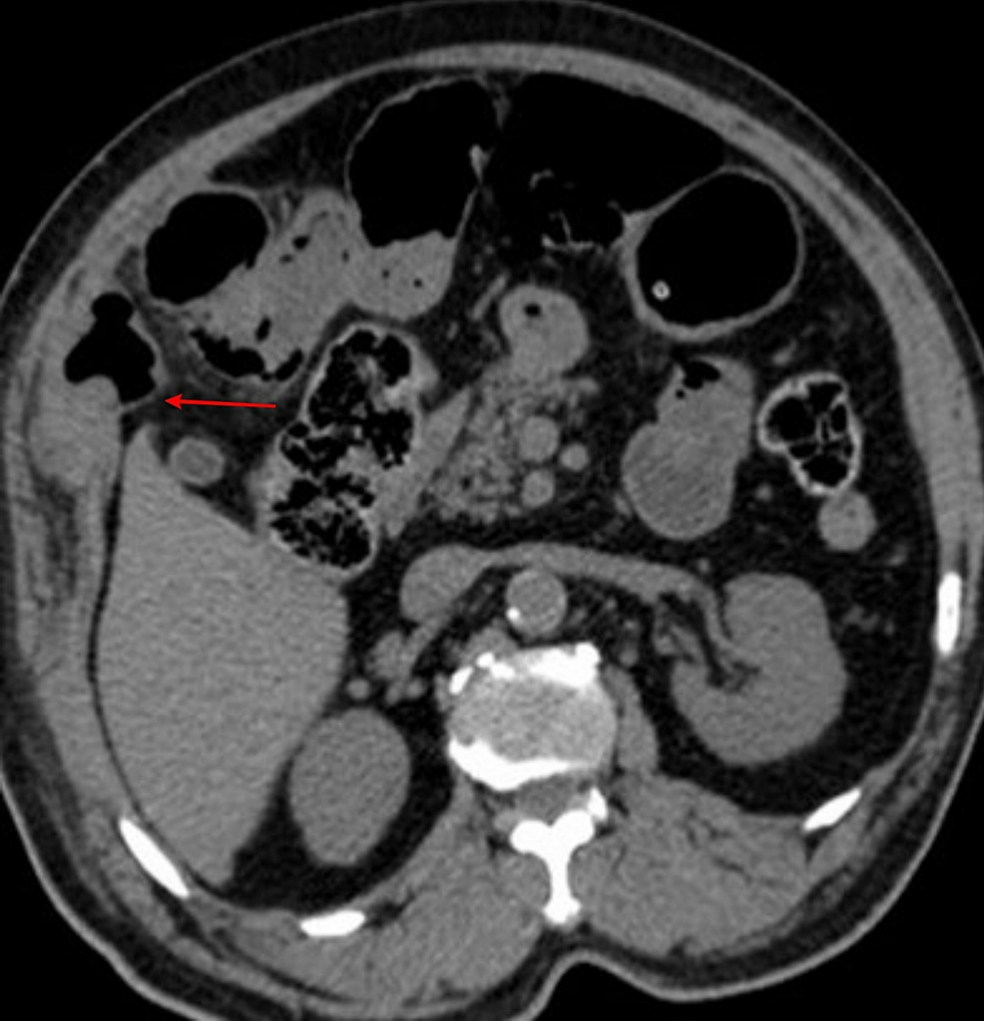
CECT of the abdomen showing herniation of ileal loops anterior to the liver (red arrow). CECT, contrast-enhanced CT
